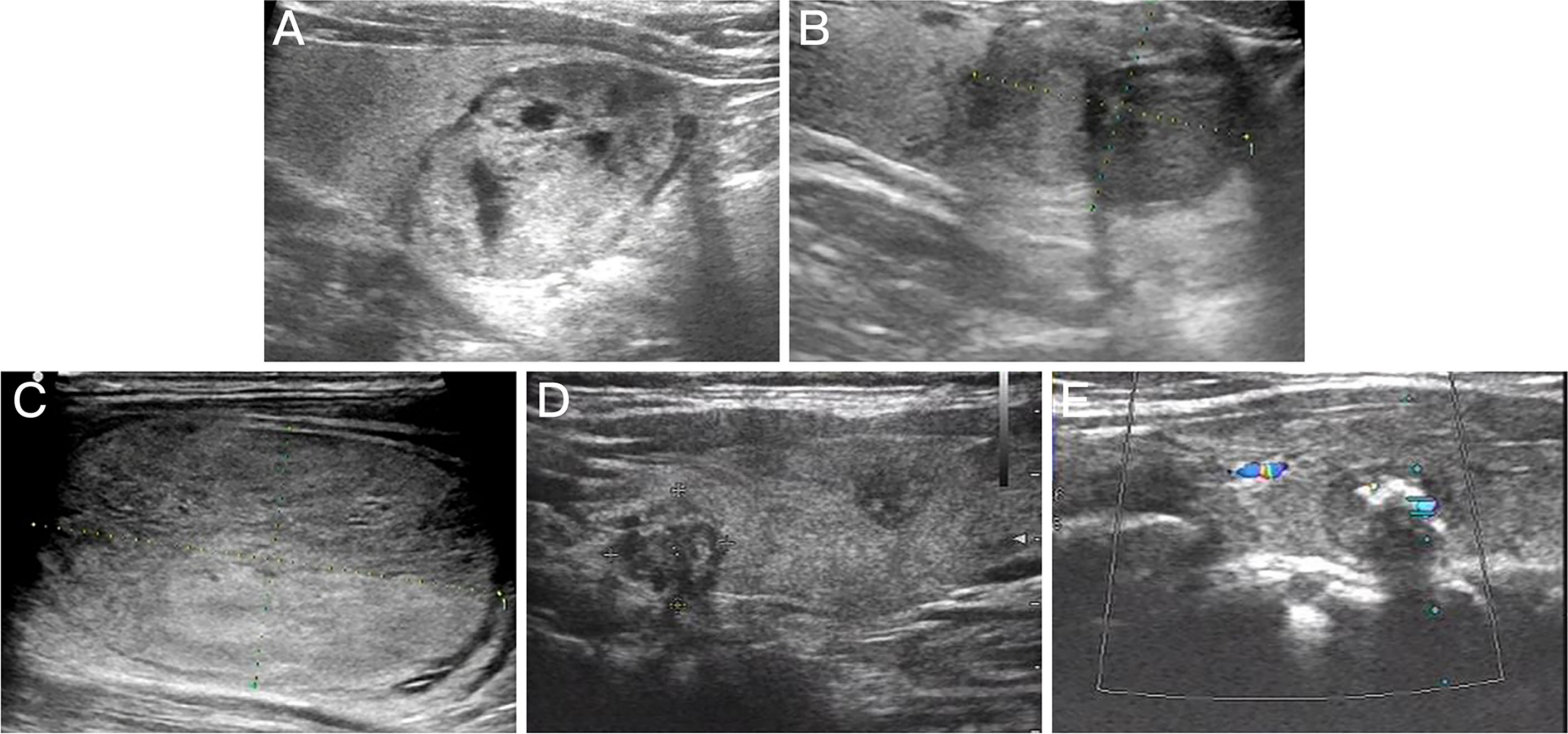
Preoperative ultrasound images for thyroid nodules with different pathologic types. (A) Follicular thyroid adenoma. (B) Follicular variant papillary thyroid carcinoma. (C) Follicular thyroid carcinoma. (D) Multifocal papillary thyroid carcinoma. (E) medullary carcinoma.
